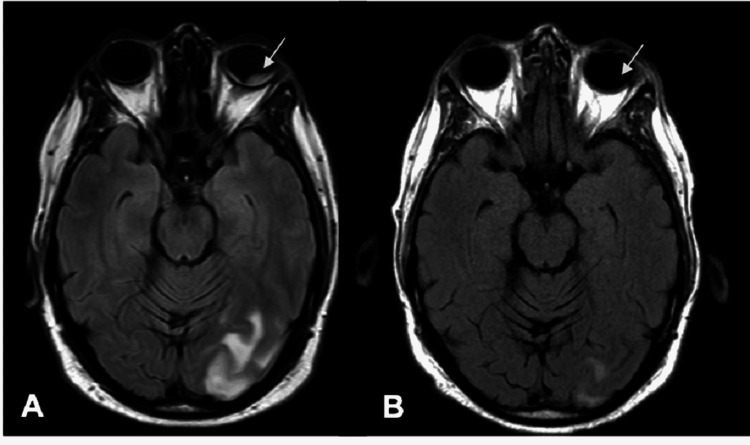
T2/FLAIR brain magnetic resonance imaging Axial images reveal a hyperintense lesion of the left posterior pole of the left globe (arrow-head) as well as left occipital lobe enhancement (A). Repeat imaging three months later shows the resolution of the left orbital mass and interval decrease in brain lesions following treatment (B). T2/FLAIR: T2-weighted fluid-attenuated inversion recovery
